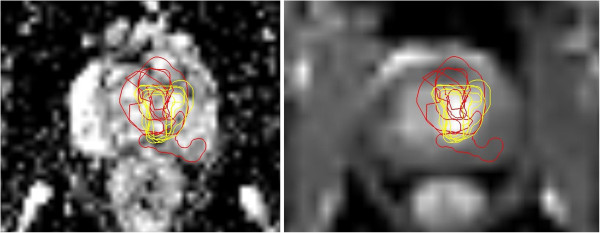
(Patient No. 3). Transversal slices through the middle of the prostate; left = DWI-, right = DCE-sequence. Left: DWI based GTV-delineation (red contours). Right: DCE based GTV-delineation (yellow contours). T2w-GTV-contours are not depicted for better survey.
